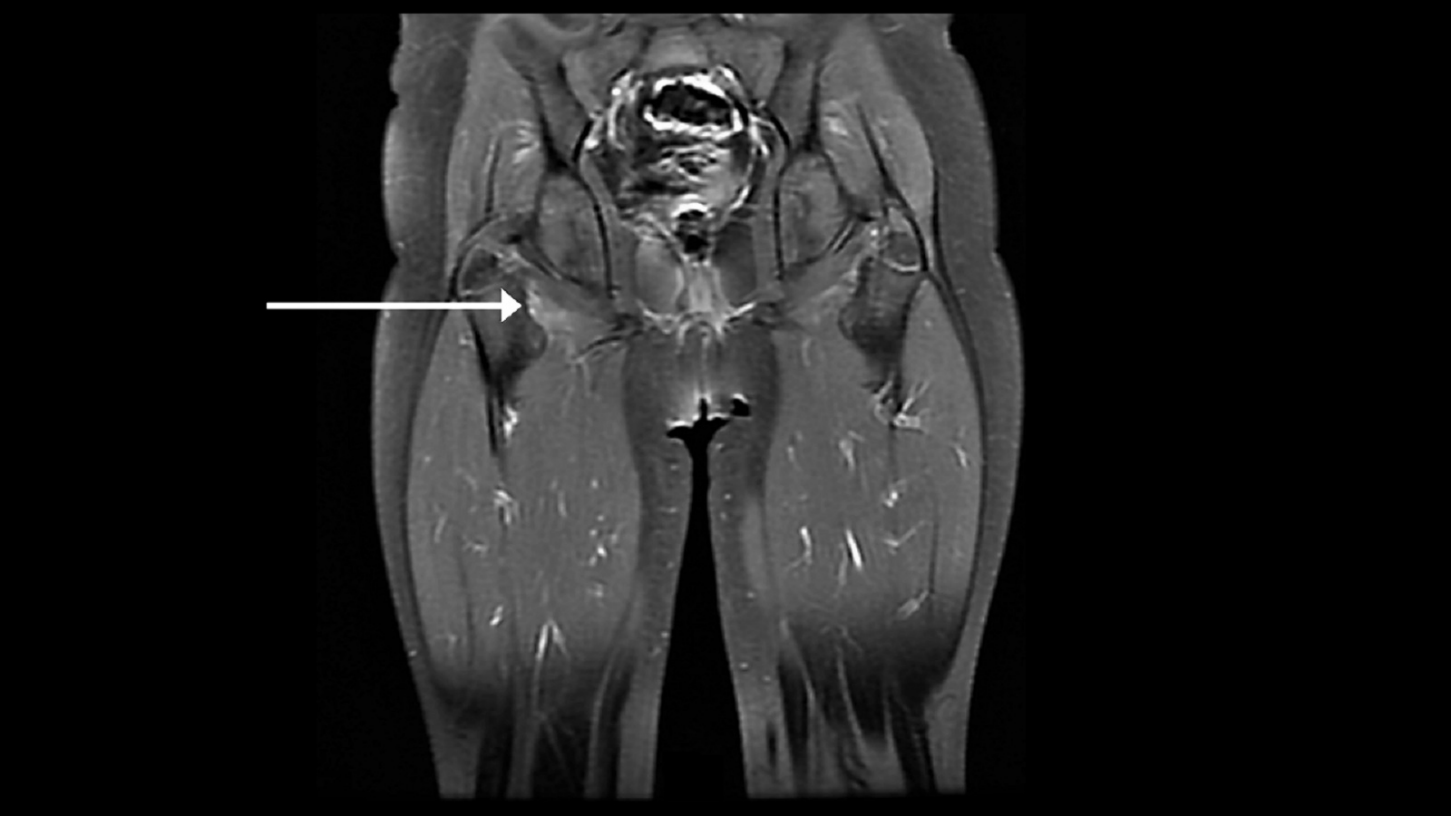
Subtle enhancement at the insertion of the right obturator externus muscle on T1 post-contrast magnetic resonance imaging
